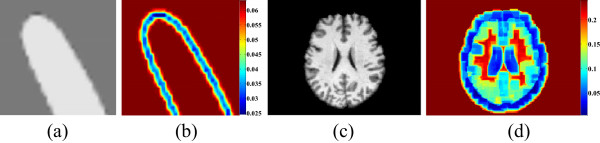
Masks of ω value. (a) Synthetic image; (b) ω value of (a); (c) MR image; (d) ω value of (c). In mask images, red to blue decreases gradually.
